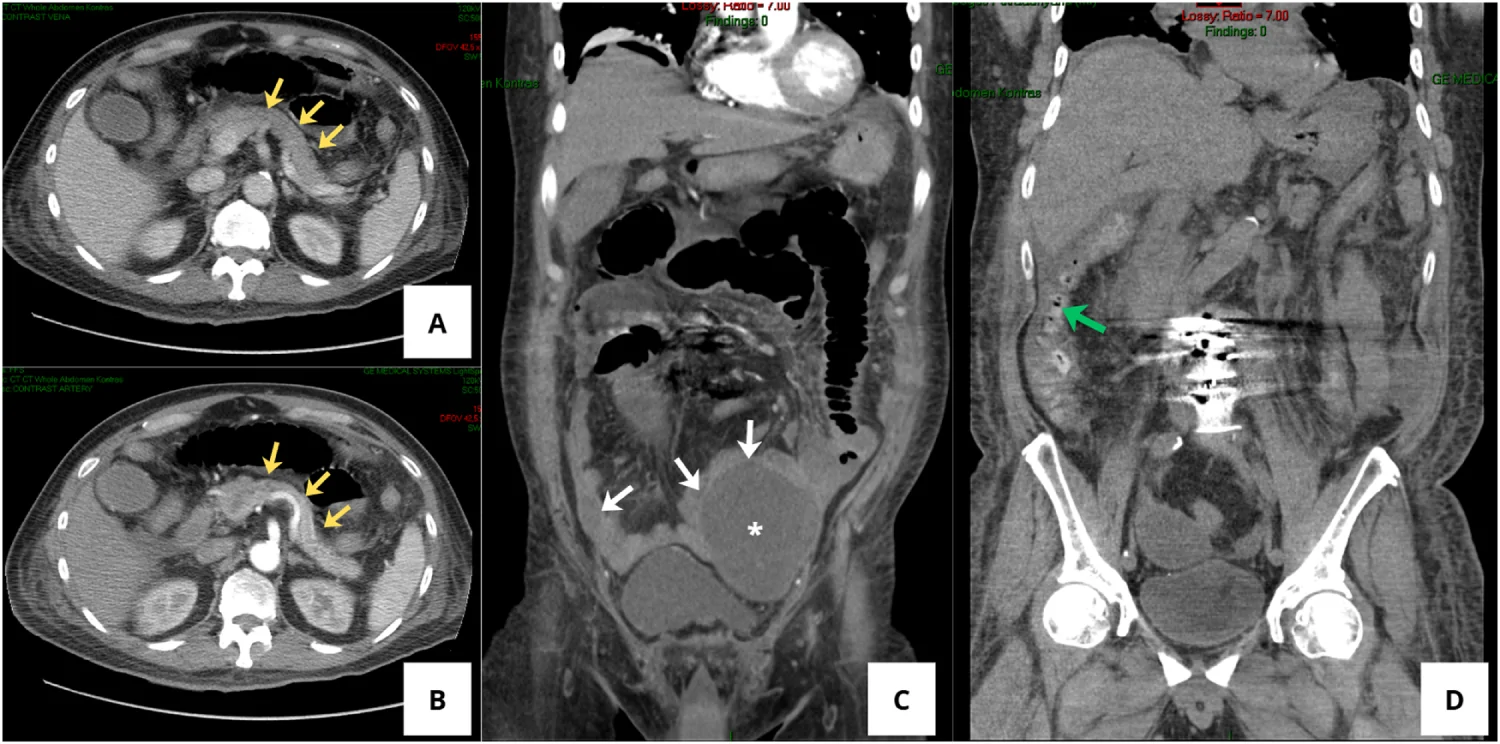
Intravenous contrast-enhanced CT scan of the abdomen showing (A and B) pancreatitis with fluid collection and peritoneal thickening (yellow arrow). (C and D) Non-contrast CT scan showing multiple walled-off pancreatic necrosis (asterisk and white arrow) in the pelvis and diverticulosis of the ascending colon (green arrow).
